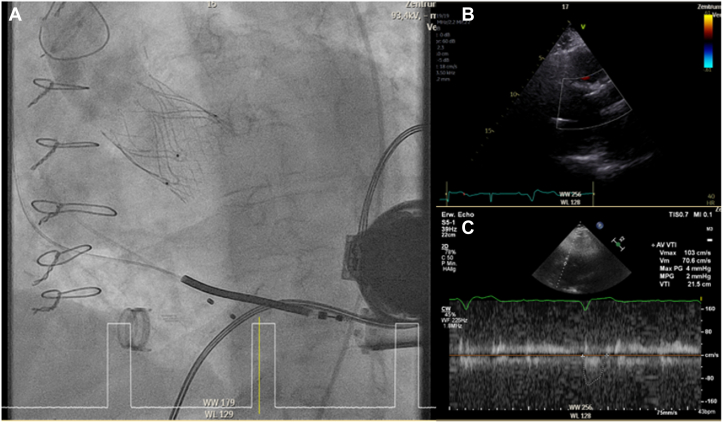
Case 2: Postimplantation Imaging (A) Postimplantation aortography. (B and C) Transthoracic echocardiography postprocedure with poorly visualized transvalvular flow on color Doppler and minimal transvalvular gradients.
